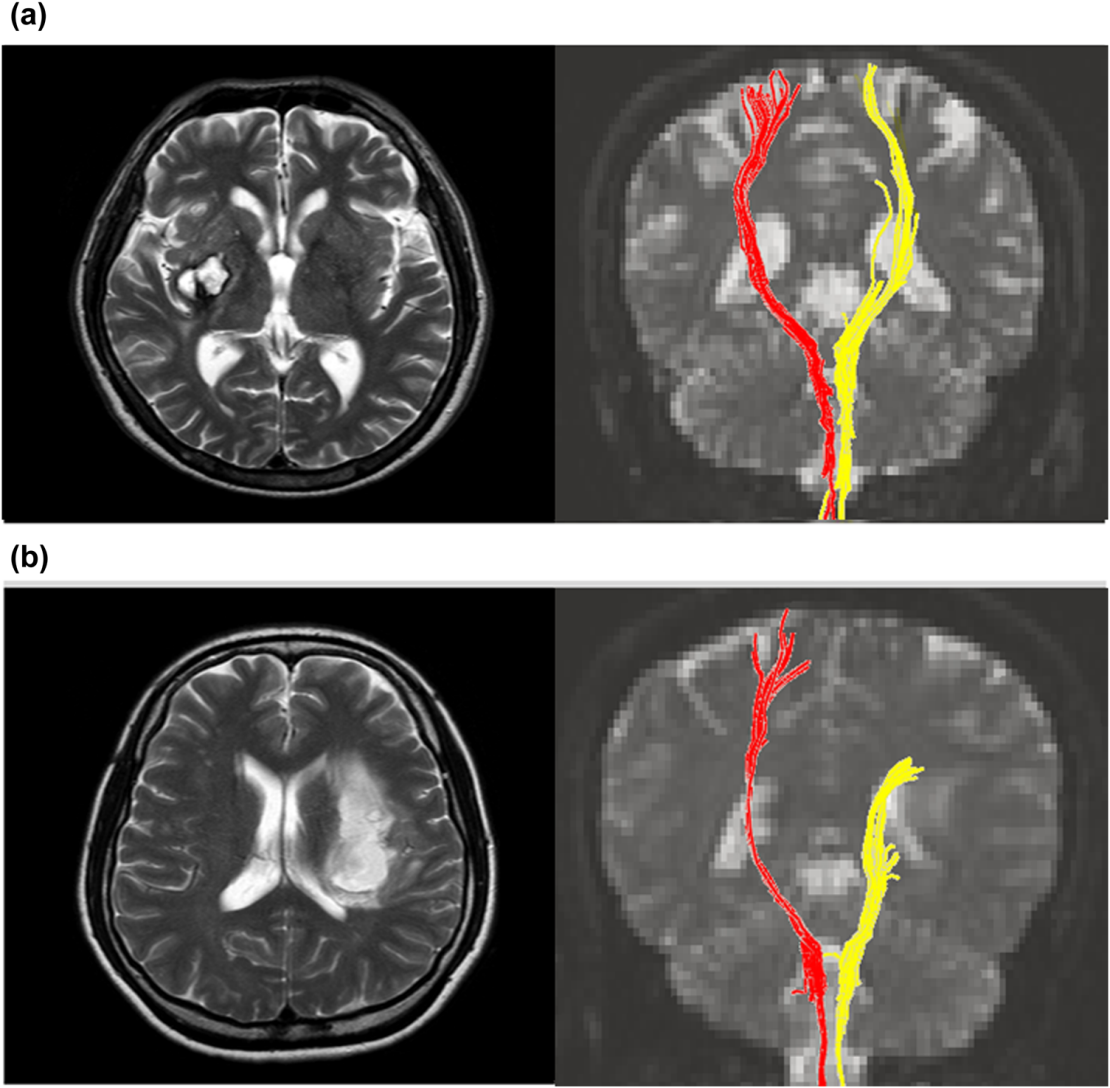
Classification according to the state of the CST on DTT. Axial *T*2-weighted magnetic resonance images (left) and coronal DTT images (right). (a) DTT+: the CST was preserved around the infarct. (b) DTT−: the CST was interrupted by the stroke lesion.
